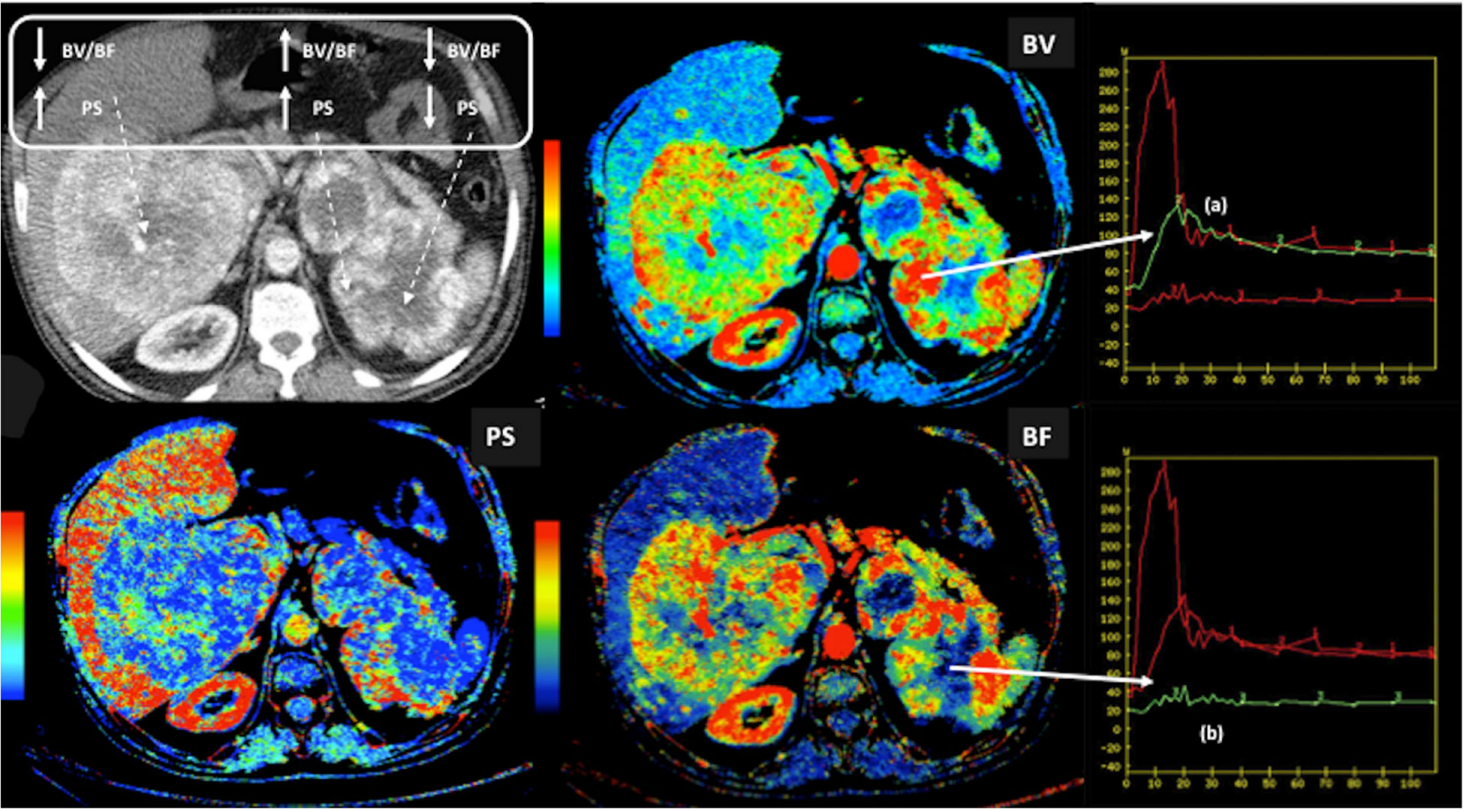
Perfusion CT exam in a 42-year-old man with a metastatic renal carcinoma. Blood flow (BF), blood volume (BV), and permeability (PS) parametric maps evidenced the heterogeneity of the functional status of the vasculature within the tumor with several combinations of the obtained parameters (top-left) and clear differences between the time density curves coresponding to the peripheral areas of viable tumor with increased BV, BF, and PS (a) with a wash-in/wash-out pattern and the central necrotic necrotic areas (a)
